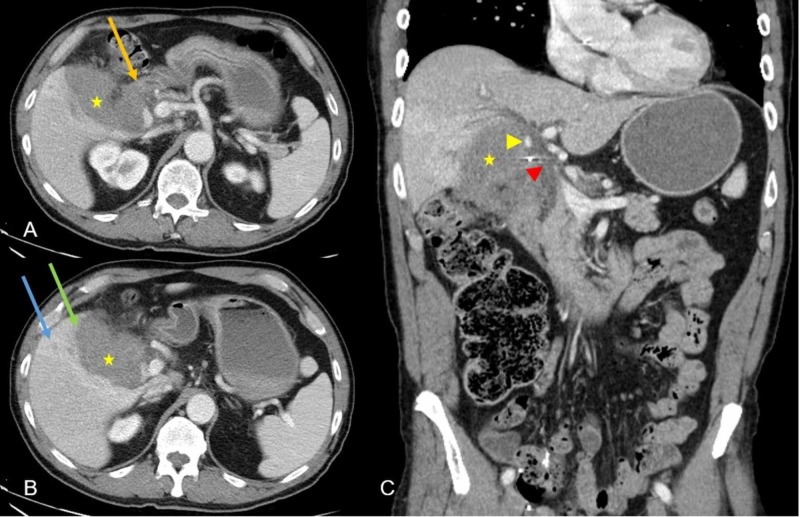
Mass in the gallbladder fossa. Multiple abdominal CT images: axial images (A, B) and coronal image (C) showing a lobulated, infiltrative, heterogeneously enhancing mass (yellow star) centered in the gallbladder fossa. Image (A) demonstrates loss of fat plane with proximal duodenum (yellow arrow). Image (B) demonstrates loss of fat plane with the inferior border of the right hepatic lobe (green arrow) and intrahepatic biliary dilation (blue arrow). Image (C) demonstrates compression and loss of fat plane with CBD (red arrowhead) and encasement of the right hepatic artery (yellow arrowhead). Hyperdense cholecystectomy clip is noted just above the red arrowhead. CBD, common bile duct
